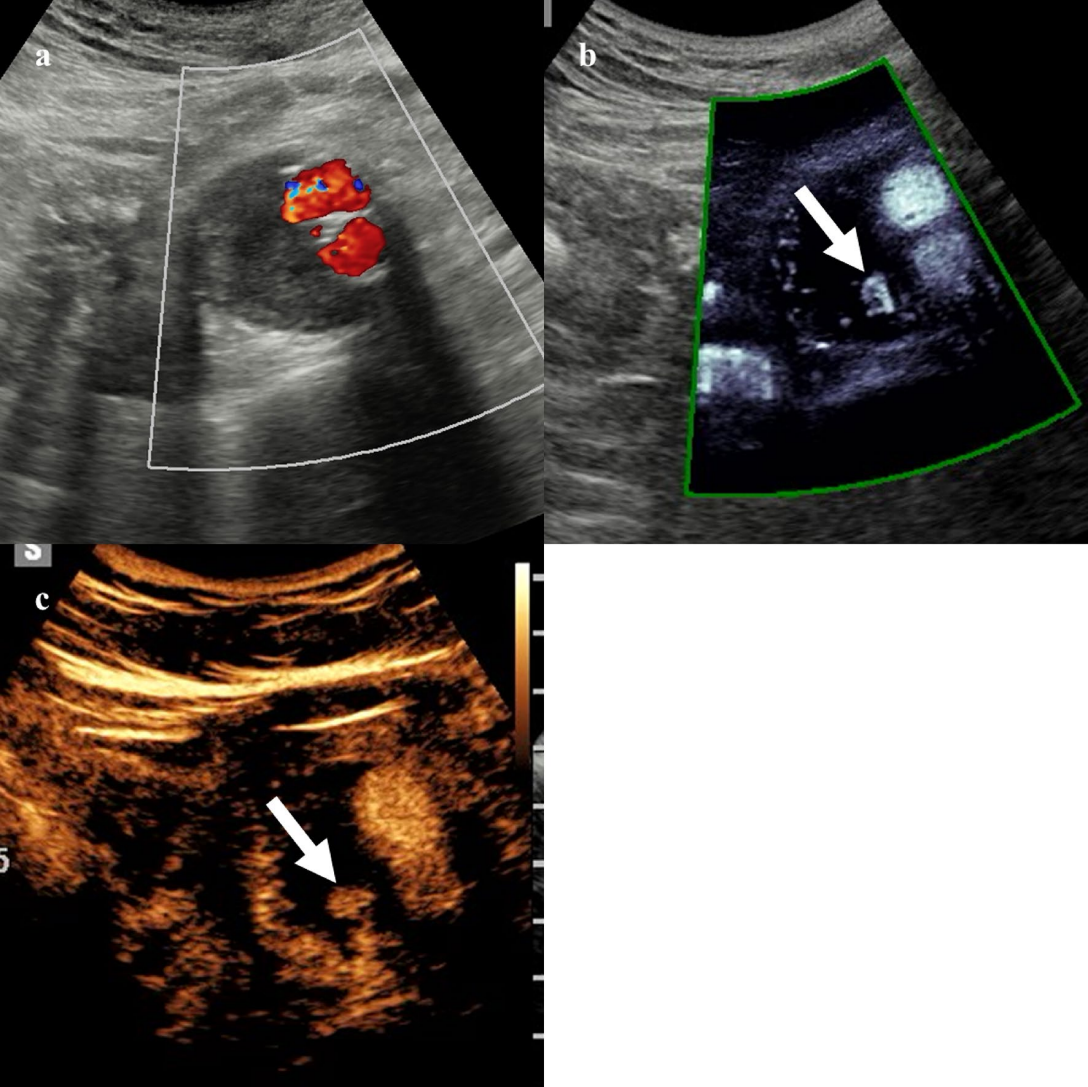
76-year-old man undergoing follow-up examination after endovascular aneurysm repair. Ultrasound examination with color doppler imaging (a) shows patency of the graft without signs of endoleak. Microvascular flow imaging (b) demonstrates the presence of a peripherally located endoleak (arrow), consistent with type II endoleak from a lumbar artery, which was confirmed on contrast-enhanced ultrasound (c, arrow, at 96 s after contrast injection)
